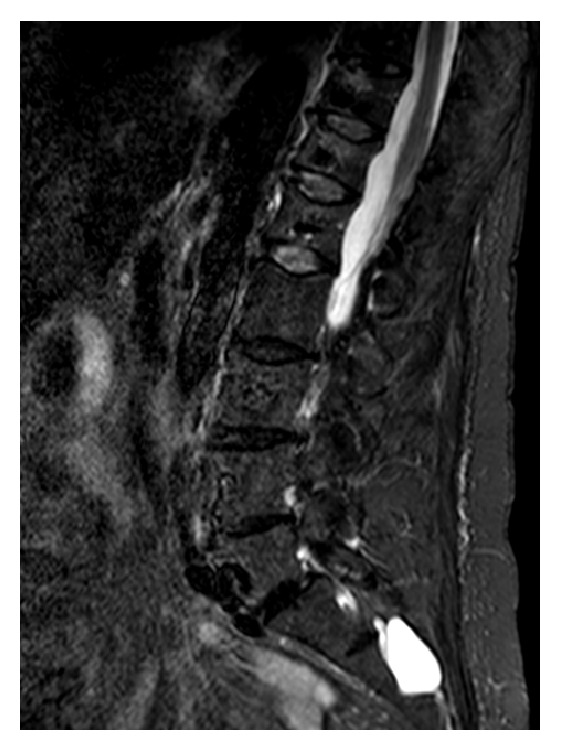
Postoperative sagittal magnetic resonance image of the spine 3 months after surgery, showing increased signal and signs of slight recollapsing, especially in the thoracolumbar transition on the STIR sequence.
